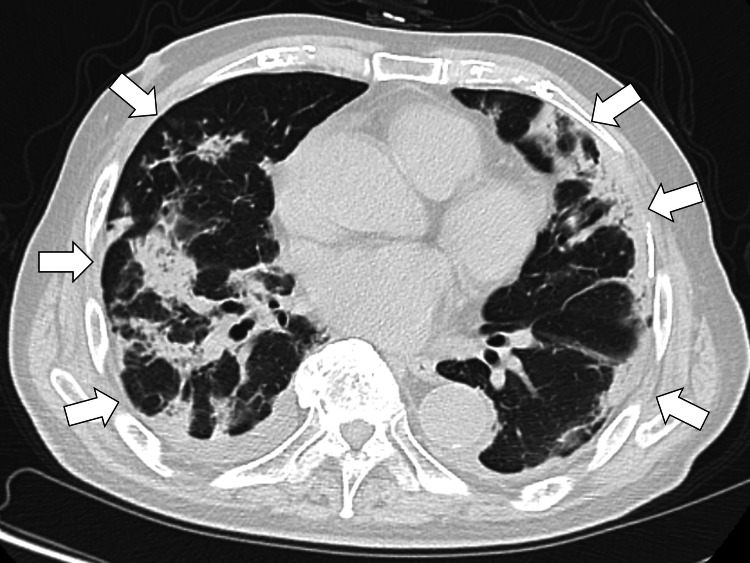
Chest CT showing multiple infiltrative opacities predominantly distributed in the subpleural regions and along the bronchovascular bundles, accompanied by bronchiectatic changes and small bilateral pleural effusions (white arrows) CT, computed tomography
